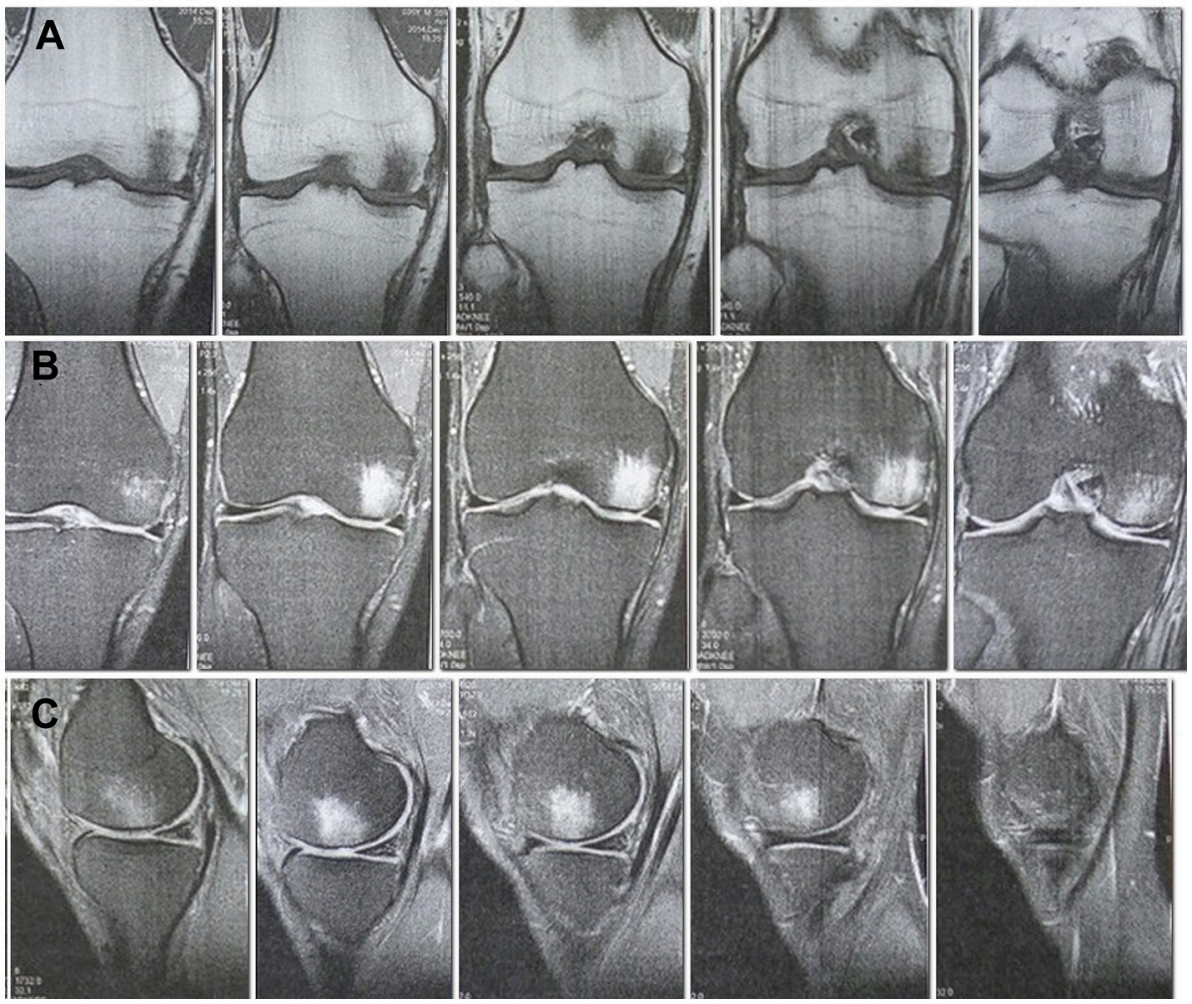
MRI of the right knee 6 months after the injury. A site of local damage to the medial condyle of the thigh is visualized. Pronounced subchondral edema. (A) Cor T1FSE. (B) Cor PD fat sat FSE. (C) Sag PD fat sat FSE.
